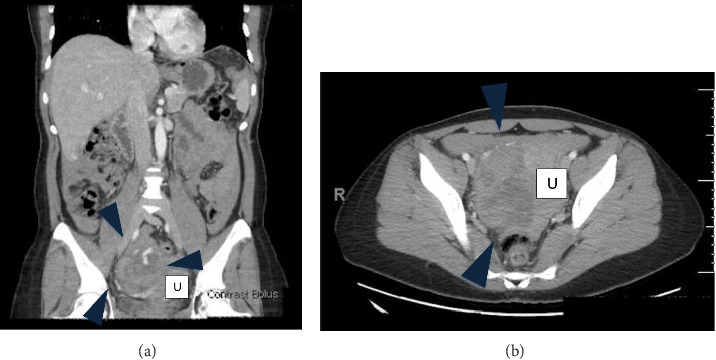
(a) Coronal view of CT showing 8.8 × 6.0 × 8.3-cm right heterogenous density mass with prominent vascularity (indicated by the filled blue arrowheads; U represents the uterus). Small to moderate amount of complex hyperdense free fluid in the abdomen and pelvis. (b) Axial view of CT showing same findings as mentioned above (indicated by the blue arrowhead; U represents the uterus). The mass is also noted to cause mass effect with deviation of the uterus toward the left.
